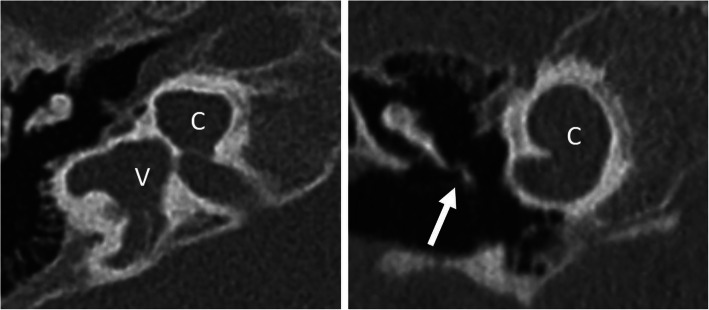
A 1-year-old male patient, with sensorineural deafness from birth. HRCT axial image (left) and coronal image (right) show incomplete partition type I, with empty cystic cochlea (C) and a large dilated vestibulum (V). Stapes is malformed and fused with the incus (arrow)
